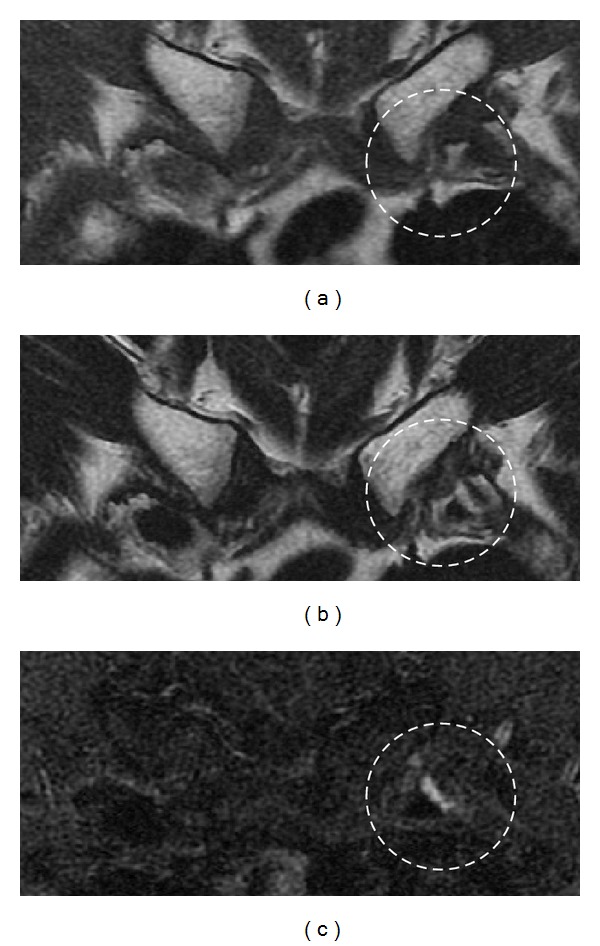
Magnetic resonance imaging of the bilateral first sternocostal synchondrosis. Coronal T1-weighted image (a), T2-weighted image (b), and STIR image (c) are shown. STIR image showing a high-intensity lesion at the left first sternocostal synchondrosis.
